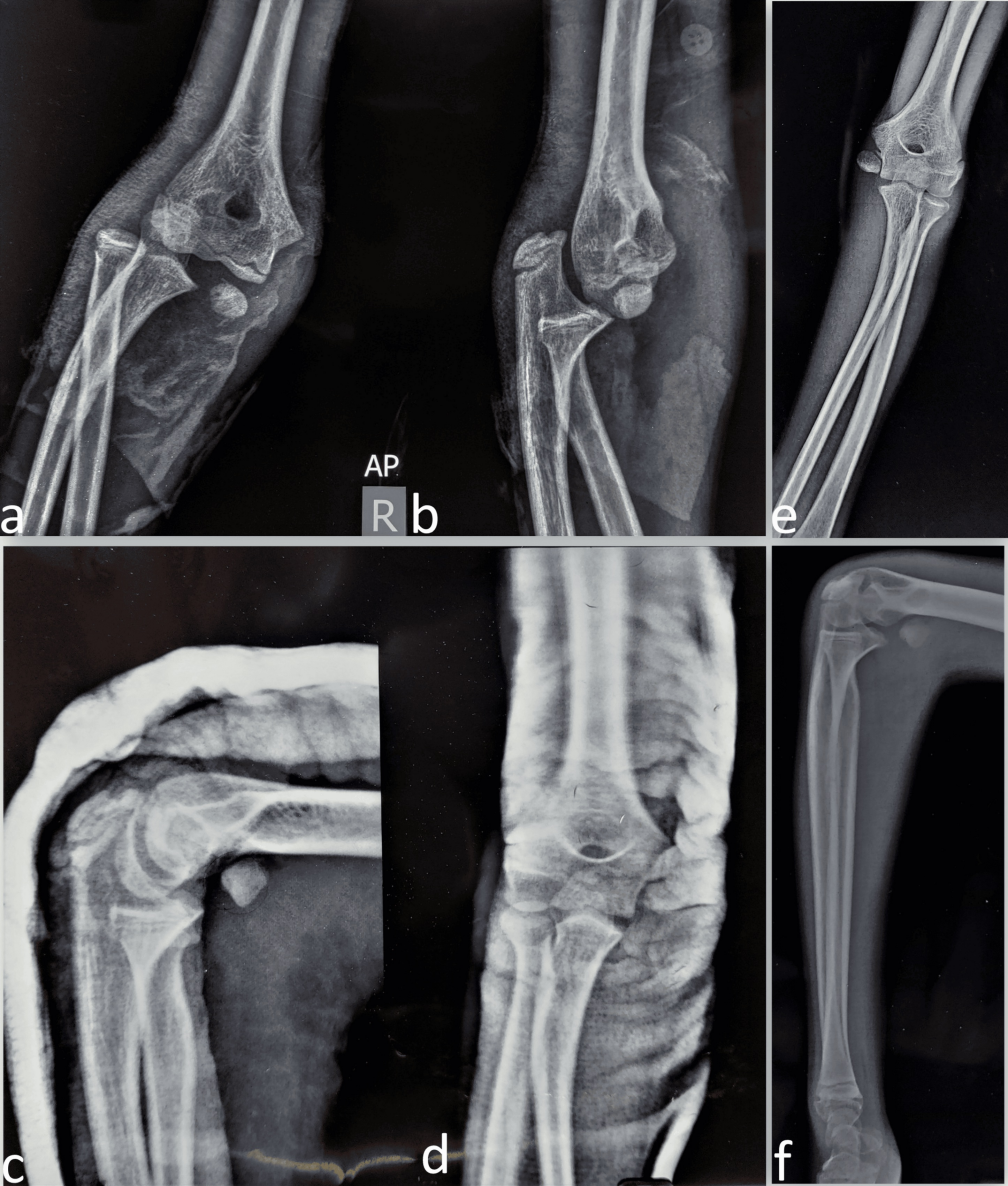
The previous radiographs show posterolateral elbow dislocation in the anteroposterior (a) and lateral (b) views. There is an associated medial epicondyle displaced fragment. The elbow dislocation was reduced well and radiographs show reduced elbow (c) given a plaster slab (d). The untreated medial epicondyle fracture is visible in the radiograph (e) after one month of the injury (f).
